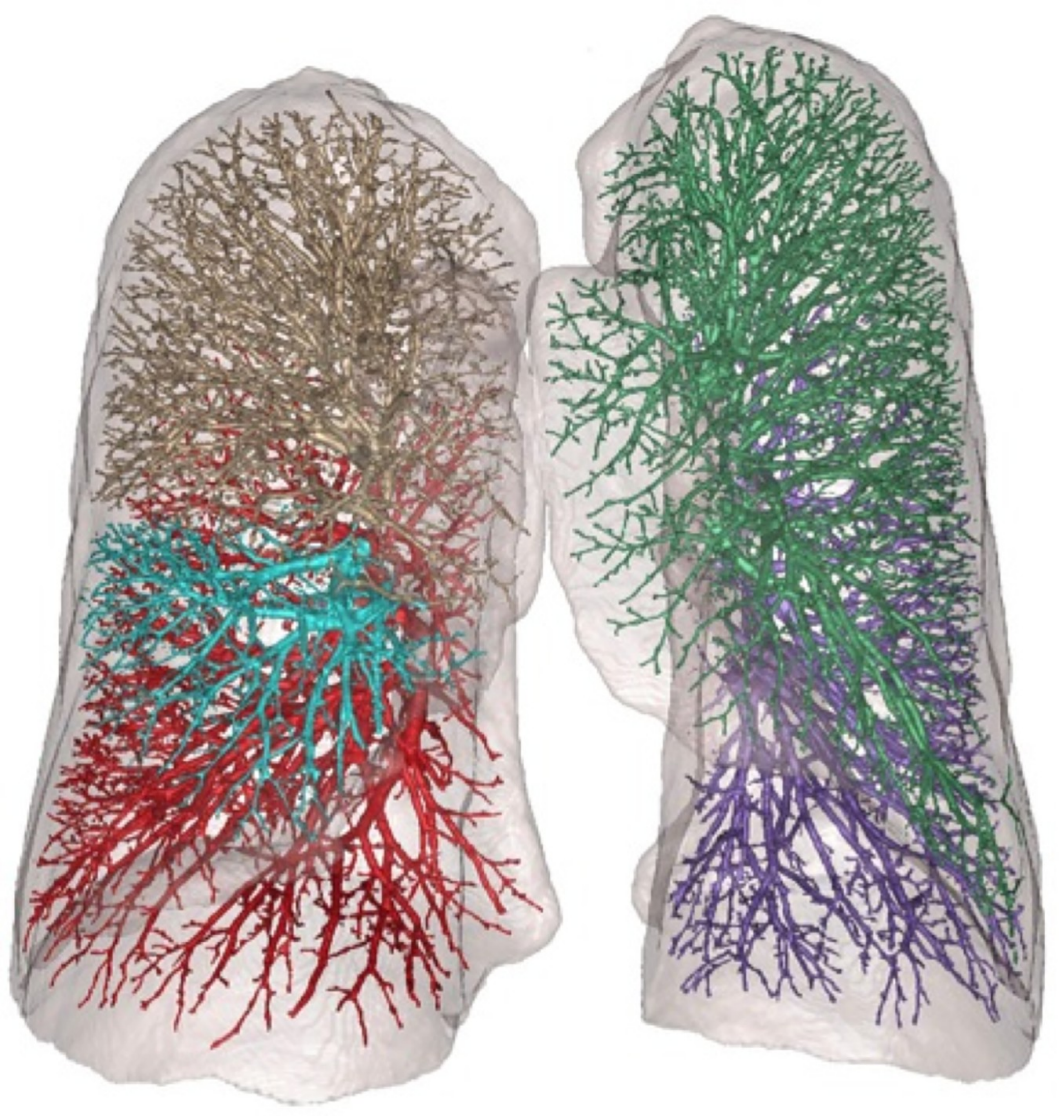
3-dimensional reconstruction of the vessels comprising the total pulmonary vascular volume and the lungs on CT scan. Colors depict the 5 lobes of the lungs; shaded region, the lung volume.
